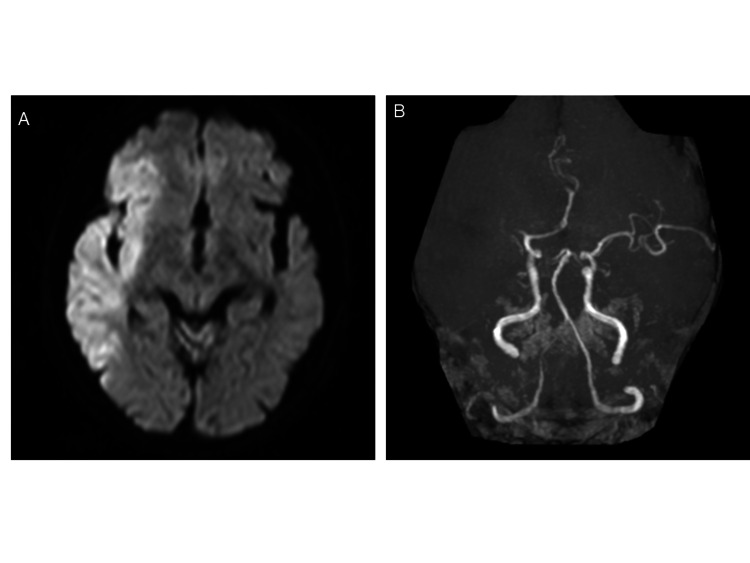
MRI obtained on initial patient admission. (A) Diffusion-weighted imaging revealed an acute ischemic stroke in close proximity of the right middle cerebral artery. DWI- Alberta Stroke Program Early CT Score was 5. (B) Magnetic resonance angiography showed a right M1 occlusion.
